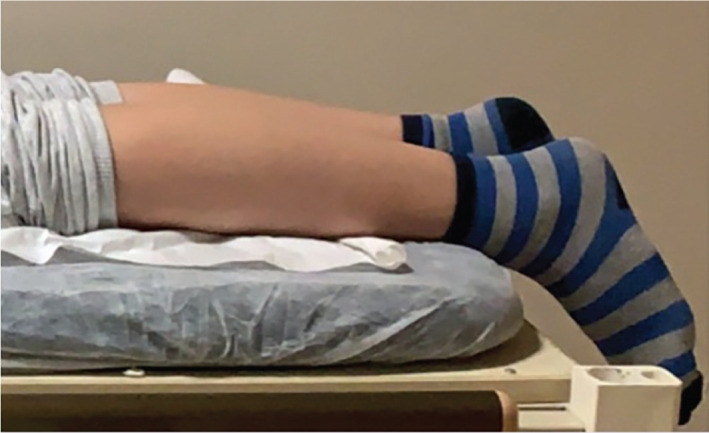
The position of the patient on the examination table while performing ultrasound measurements.
